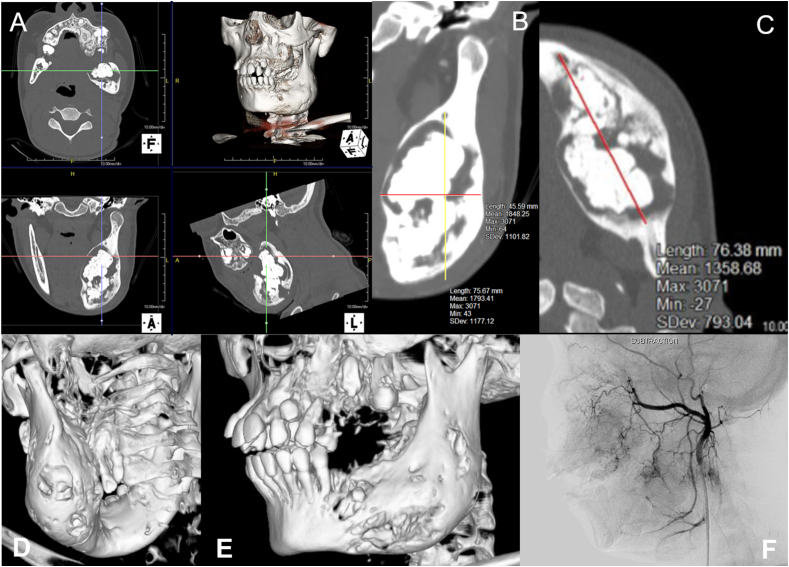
Preoperative findings A,B,C: Preoperative computed tomography image showing the size of the mandibular tumor. D,E: Preoperative 3-dimensional computed tomography image showing significant enlargement of the mandibular tumor. F: Angiographic computed tomography image at the time of embolization, showing dilated peripheral blood vessels and abundant blood flow into the lesion.
